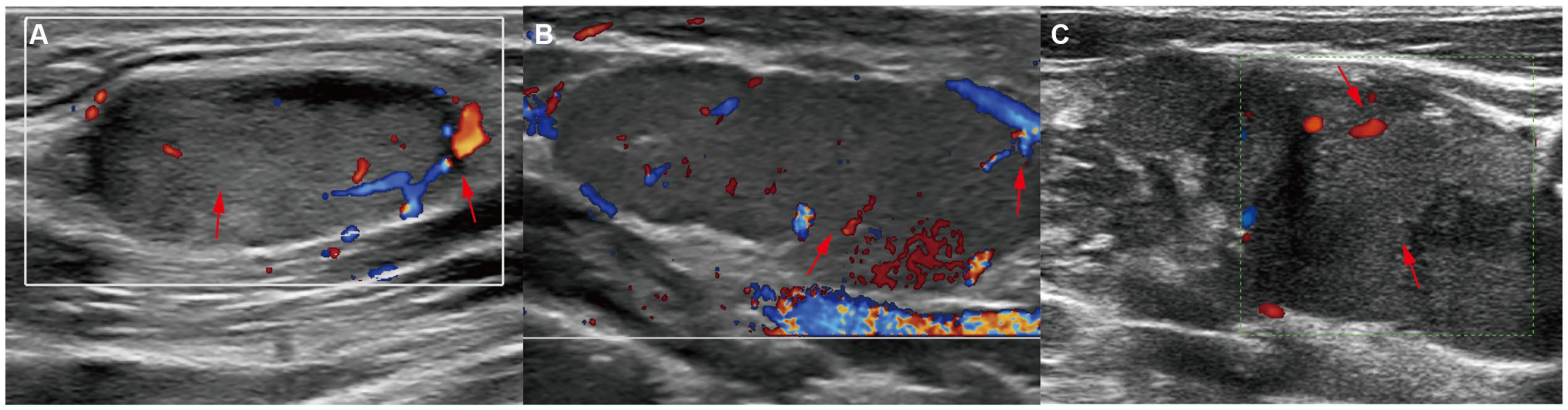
Ultrasound features of absent hila. CDFI of (A) left cervical LN from a 29-year-old patient, (B) left cervical LN from a 32-year-old patient, and (C) right cervical LN from a 37-year-old patient showed the absence of hila and peripheral-type blood flow signals (red arrows).
